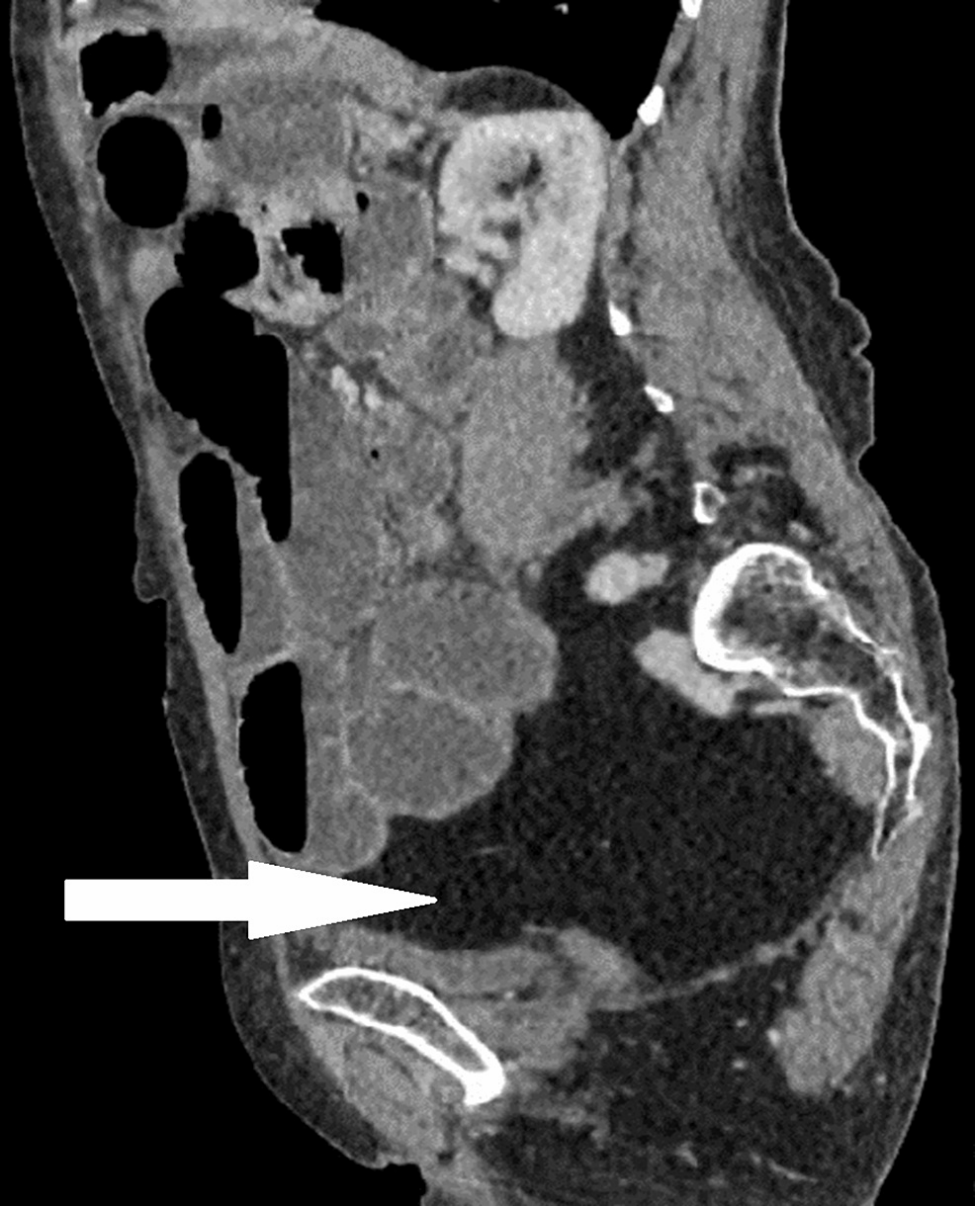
Axial view of left-posterior intra-pelvic lipoma on computed tomography of the abdomen and pelvis.
